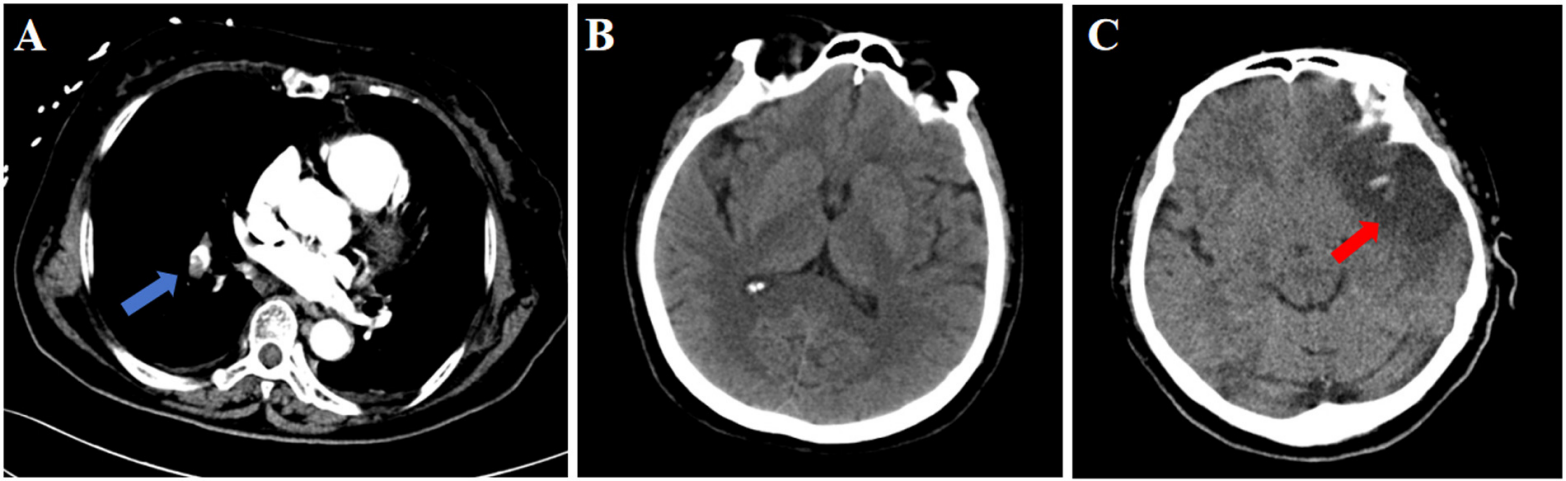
CT findings of the patient. (A) Pulmonary CT on postoperative day 1; (B). Cranial CT on postoperative day 1; (C). Cranial CT on postoperative day 3. Blue arrow: pulmonary embolism; Red arrow: stroke.
